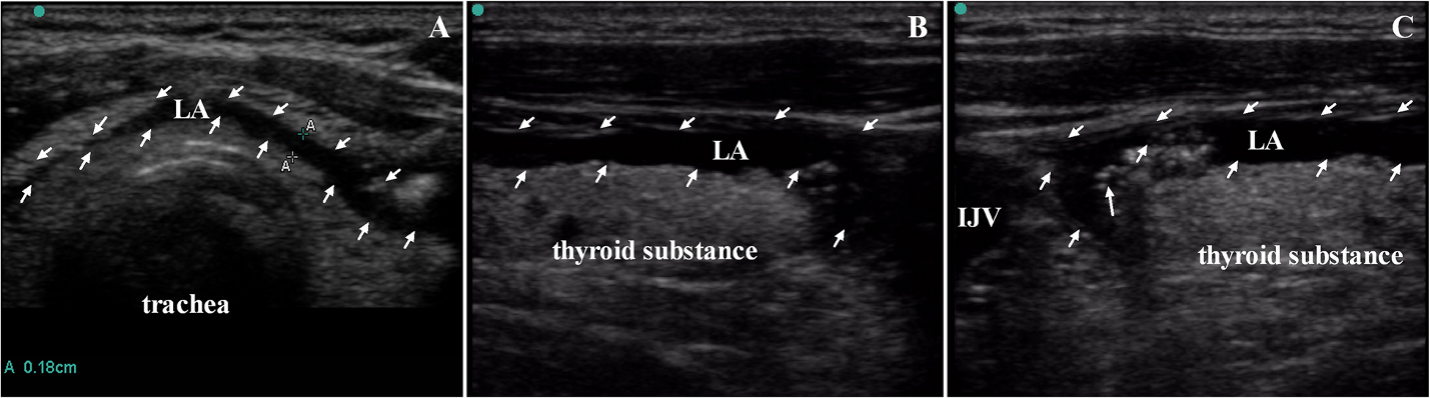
Typical ultrasonogram of the distribution of local anesthetics after ultrasound-guided capsule-sheath space block (CSSB) was performed in a 38-yr-old female patient. (A) Sonogram of the thyroid gland in the transverse plane showing the uniform distribution of local anesthetics solution thickness 0.18 cm; (B) Sonogram of upper pole of the thyroid gland in the longitudinal plane showing the uniform local anesthetics solution around thyroid; (C) Sonogram of lower pole of the thyroid gland in the longitudinal plane. IJV, internal jugular vein; LA, local anesthetics; SCM, sternocleidomastoid muscle.
